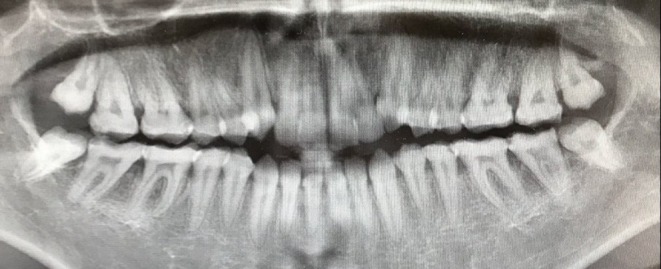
Preoperative orthopantomogram showing almost no enamel surrounding molars.
